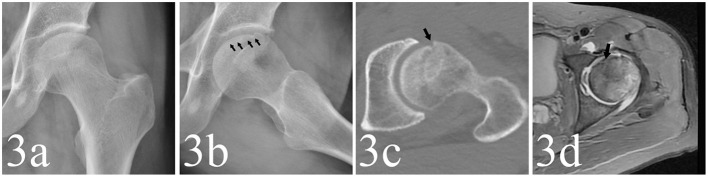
(a,b) A 48-year-old male patient whose left hip appeared to be collapsed in FL view (as the black arrows point) but not in AP view. (c,d) The CT and MRI detected the collapse in anterior femoral head (as the black arrows point).
